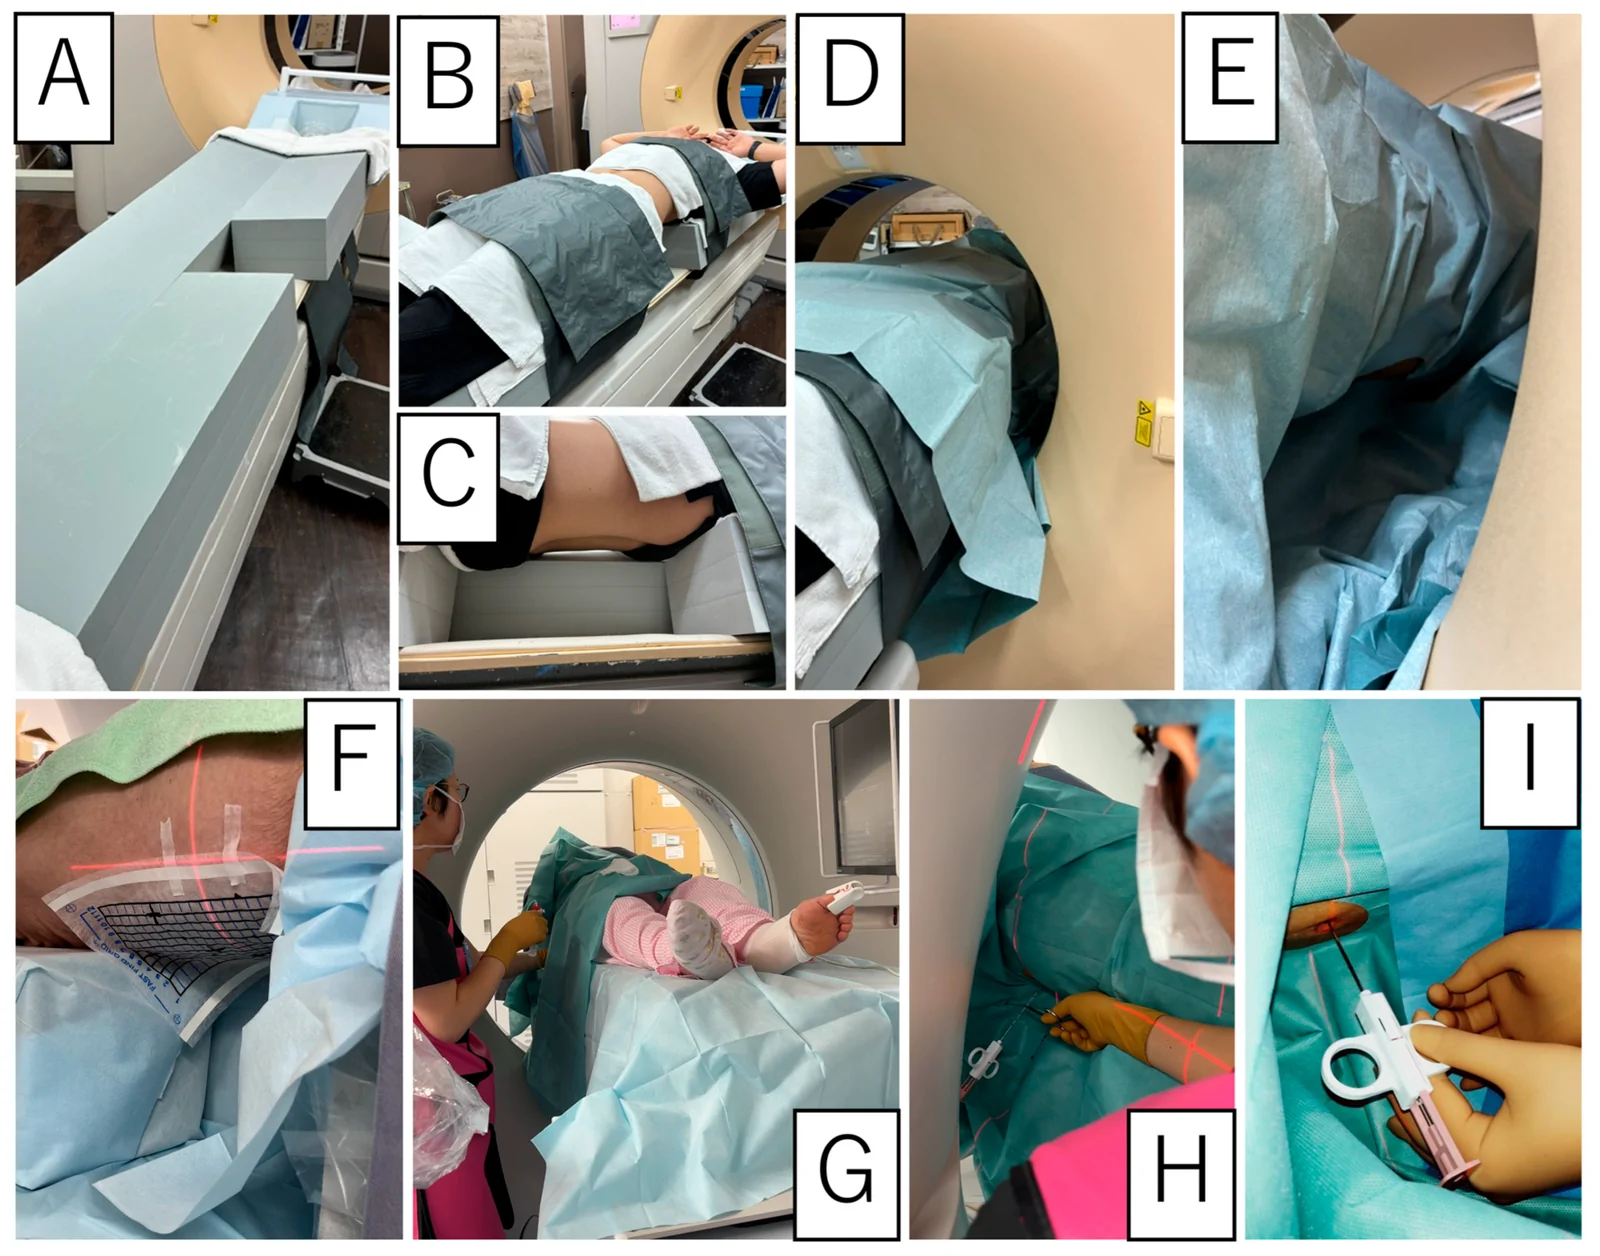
Patient positioning, dorsal space setup, and ergonomics of the upward approach. (A) Layout of foam mats on the CT couch: Three hard foam mats are arranged on the CT table to create the dorsal working space. The position and extent of the dorsal space can be adjusted by altering the distance between the mats. (B) Volunteer positioning and safety restraint: A healthy volunteer positioned on the mats. Routine safety belts attached to the CT couch are secured across the patient. Because the head, shoulders, buttocks, and lower extremities are supported by the two mats, the patient’s posture remains stable despite the unsupported dorsal space. (C) Close-up of the created dorsal space: The setup provides a working space measuring 30–45 cm in width, 15 cm in height (using a 15 cm mat), and 22 cm in depth. (D) Positioning within the CT gantry: The draped subject inside the CT gantry. The maximum elevation height is restricted by the gantry aperture. Care is taken to prevent contact between the gantry and the patient’s face or upper limbs during table movement. (E) Ergonomic operator hand placement: Close-up of the draped dorsal space demonstrating hand positioning. Resting the operator’s hand/forearm directly on the CT couch provides a stable pivot and minimizes hand fatigue during upward insertion. (F) Skin marking in a clinical procedure: Laser marking for entry point localization in an actual patient. When the entry point approaches the dorsal midline, visibility of the red laser reference line decreases due to shadow effects. (G) Real-time puncture scene: Overall clinical view during needle placement using the dorsal space. (H) Needle stabilization with plastic forceps: Close-up of the hands. At shallow insertion depths, non-magnetic forceps are used to support the needle hub and prevent displacement or dislodgement during verification CT scans. (I) Upward trajectory control: Close-up showing the upward angle of needle advancement managed from underneath.
